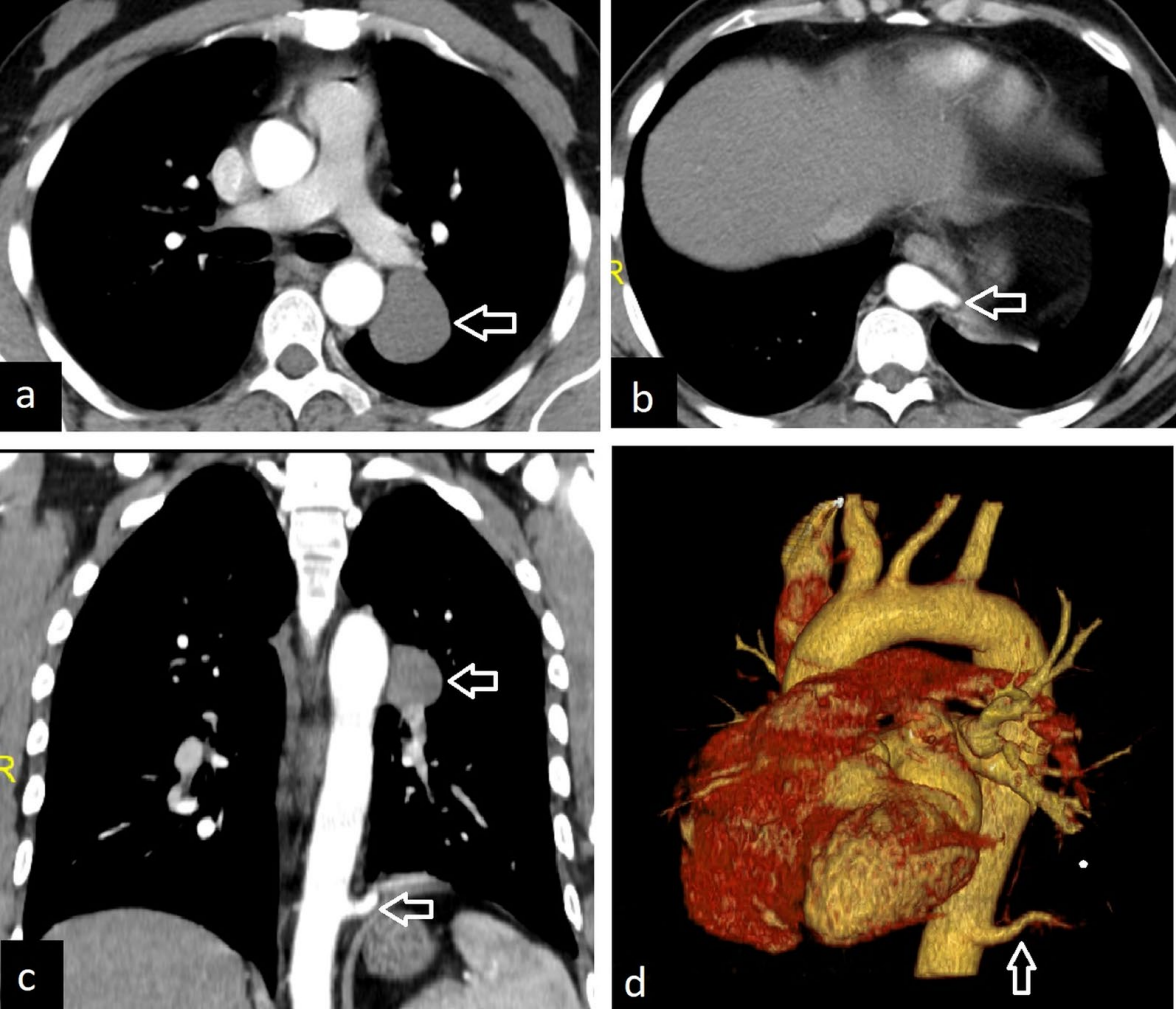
Mixed lesions-pulmonary sequestration and bronchogenic cyst in a 38-year-old male who presented with dyspnea. Contrast-enhanced axial CT images show bronchogenic cyst (a, arrow) and aberrant artery arising from the descending aorta and feeding the left lower lobe sequestration (b, arrow). Coronal CT image (c) shows both bronchial cyst and aberrant artery of sequestration (arrows). 3D volume-rendered CT image (d) shows the aberrant artery (arrow)
